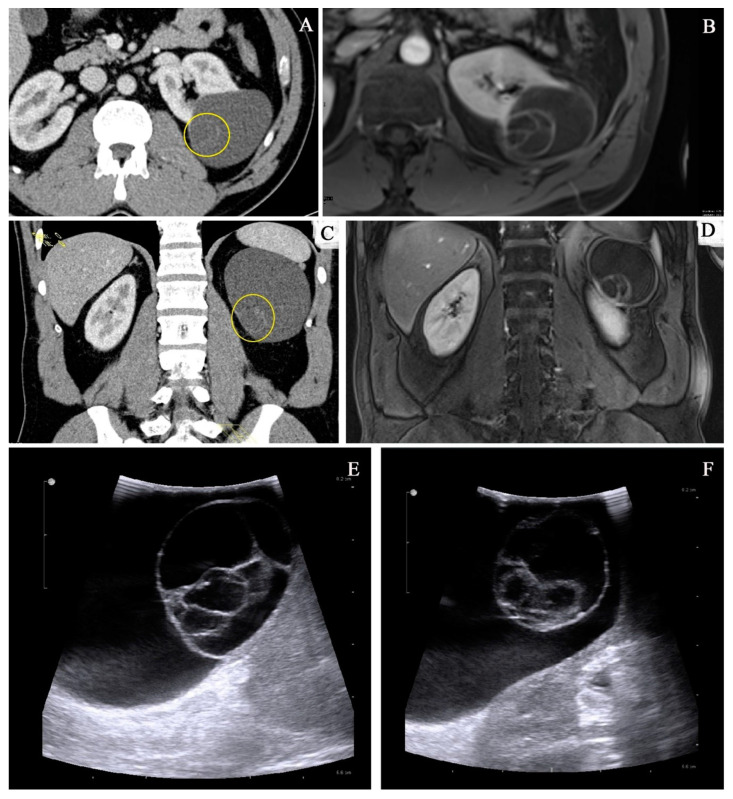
Imaging methods: comparison of CT imaging (A,C) and MRI (B,D) of the same lesion. There is a clearly visible benefit of MRI in imaging of the inner architecture with more precise imaging of the septa. (E,F) Intraoperative ultrasound image of MCRNLMP.
